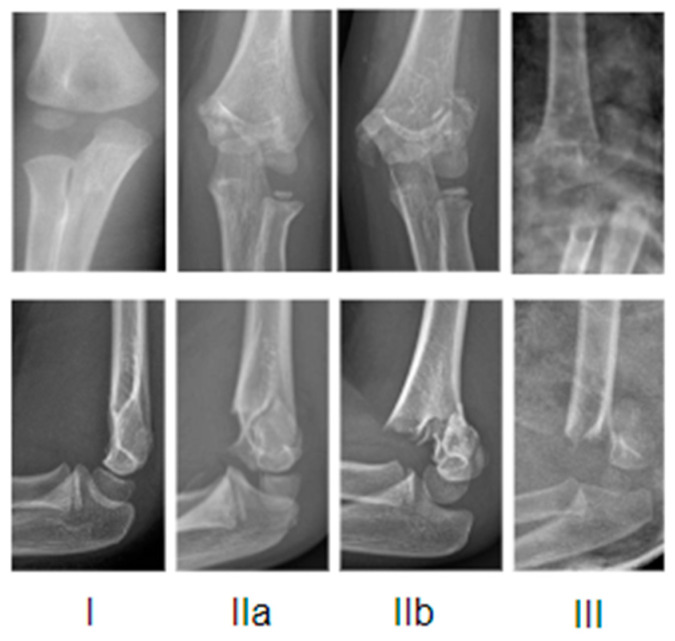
Exemplificative X-rays representation of Gartland classification.
